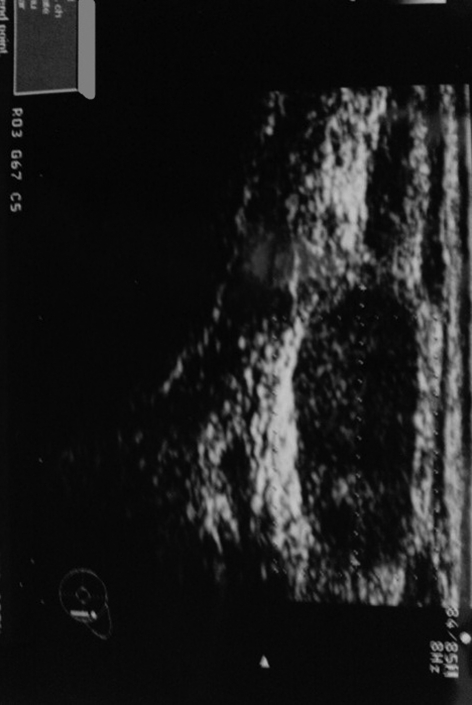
Ultrasonography shows a slightly lobulated hogogenous hypoechoic mass with slight posterior acoustic enhancement, without calcifications.
